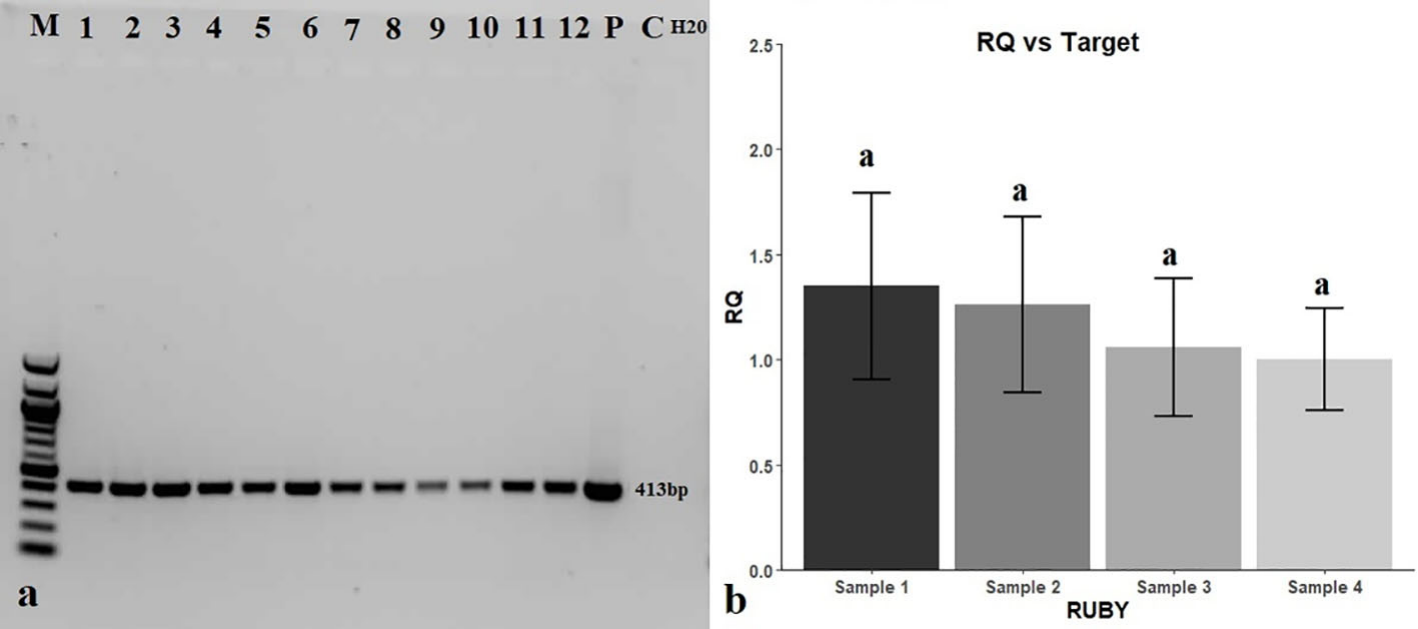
Molecular analysis of putative transgenic red hairy roots for the presence of the *bar* gene and expression of the *RUBY* gene. (A) PCR detection of the *bar* gene in transgenic hairy roots. M: DNA marker (100 bp), 1-12: red putatively transgenic hairy roots, P: 35S_Ω_RUBY plasmid as a positive control (+), C: white hairy roots as a negative control, H_2_O: negative control without template. (B) Expression of the *RUBY* reporter gene in transgenic hairy roots normalized relative to ubiquitin (Gma.441.1.S1_at) and actin (GmaAffx.90181.1.A1_at) genes using the 2^-△△CT^ method.
